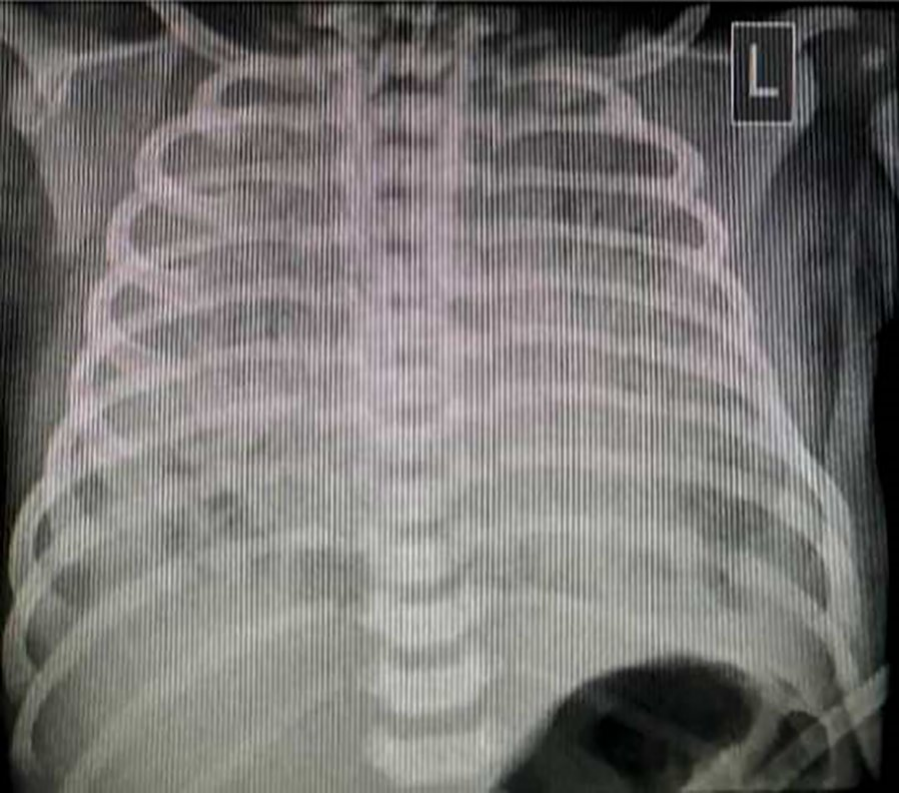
Plain radiograph of posteroanterior view in supine position, showing diffuse ground-glass opacity in both lungs
